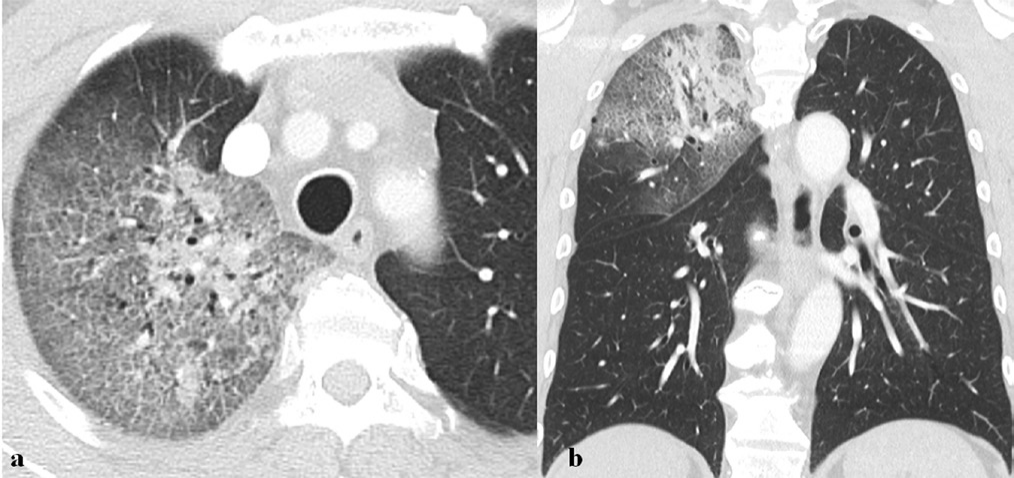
A 68-year-old male patient with MERS-CoV infection. He had a history of visiting Bahrain and suffered from fever, myalgia, and dry cough. (a, b) Axial and coronal images of chest CT scan obtained 4 days after symptom onset show mixed consolidation and ground-glass opacity with the crazy paving appearance in right upper lobe.
